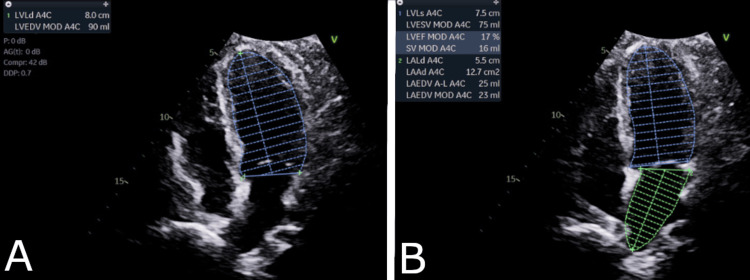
Transthoracic echocardiogram showing (A) end-diastolic frame of the left ventricle and (B) end-systolic frame of the left ventricle.
